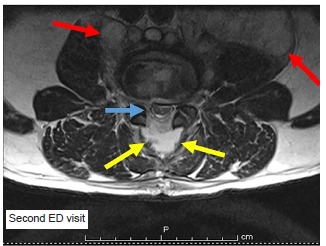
Axial magnetic resonance image at the level of the third lumbar vertebrae taken on the second visit demonstrates progression of ventral epidural abscess now extending to completely surround the lumbar nerve roots (blue arrow), and extending into posterior vertebral space (yellow arrows). Again the left psoas muscle demonstrates inflammatory changes concerning for abscess with interval progression of right psoas muscle inflammation (red arrows). *ED*, emergency department.
